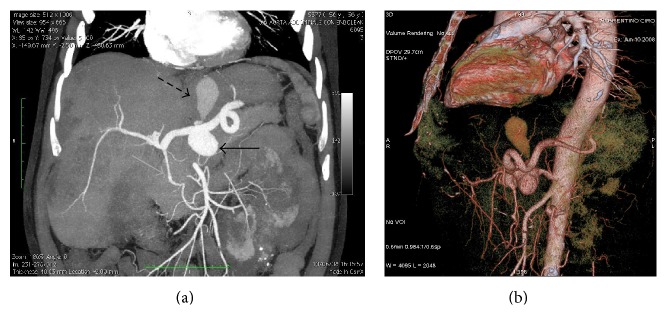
CT scan. (a) Maximum intensity projection (MIP) reconstruction of the arterial phase in the coronal plane showing a 3.8 cm aneurysm of the celiac trunk (solid black arrow) with a 4.3 cm pseudoaneurysm (dashed black arrow) originating from the dissection of the superior margin of the aneurysm sac. An ectasic pancreaticoduodenal arcade is highlighted (grey arrow). (b) Volume rendering (VR) reconstruction in the sagittal plane showing aneurysm and pseudoaneurysm.
